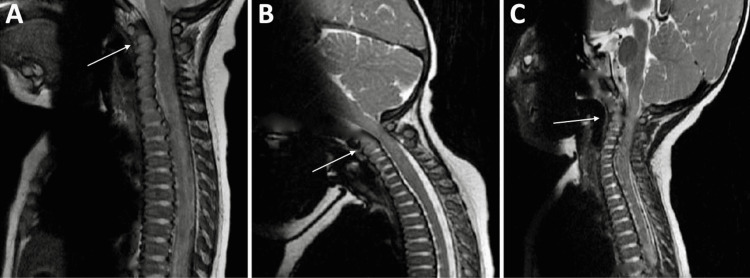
Preoperative T2 weighted MRI sagittal sequences demonstrating dynamic instability and compression of the upper cervical spinal cord on flexion and extension positioning (arrow demonstrating site of maximal compression). A: Neutral Position, B: Flexion, C: Extension.
